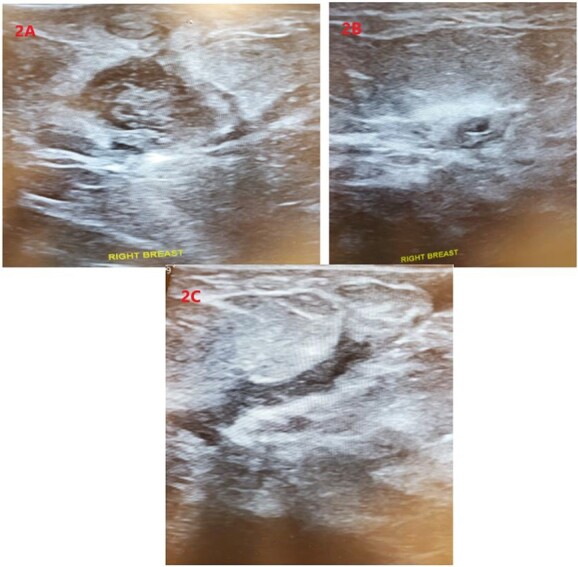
Sonographic imaging of the right breast identifying two complex fluid collections (A) and (B) at the 3:00 position. (C) Showing a fistula tract.
